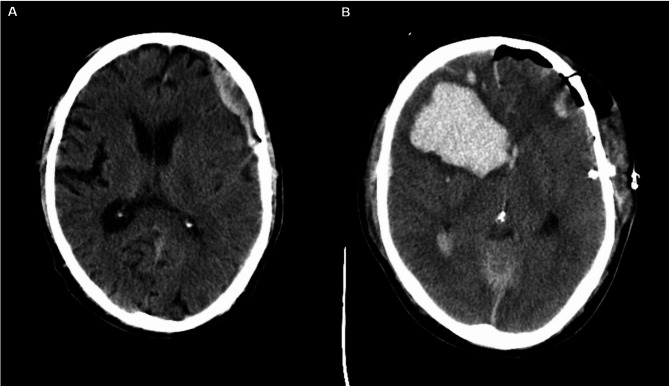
CT scan of traumatic brain injury with subdural hematoma before (A) and after (B) surgical evacuation. Although the good clinical state and equipose situation during surgery allowed for the evacuation via craniotomy, postoperative intracranial hemorrhage occured postoperatively. Notably, no prophylactic anticoagulation had been administered yet.
